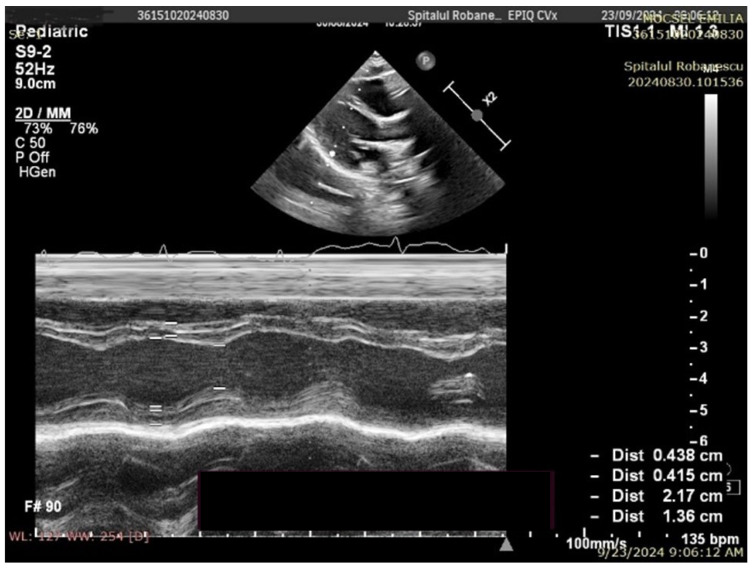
Transthoracic echocardiography in an SMA type I patient (8 months old, 7.4 kg)—M-mode measurements (LVM = 14.24 g, *p* = 0.05%, Z score −3.27).
